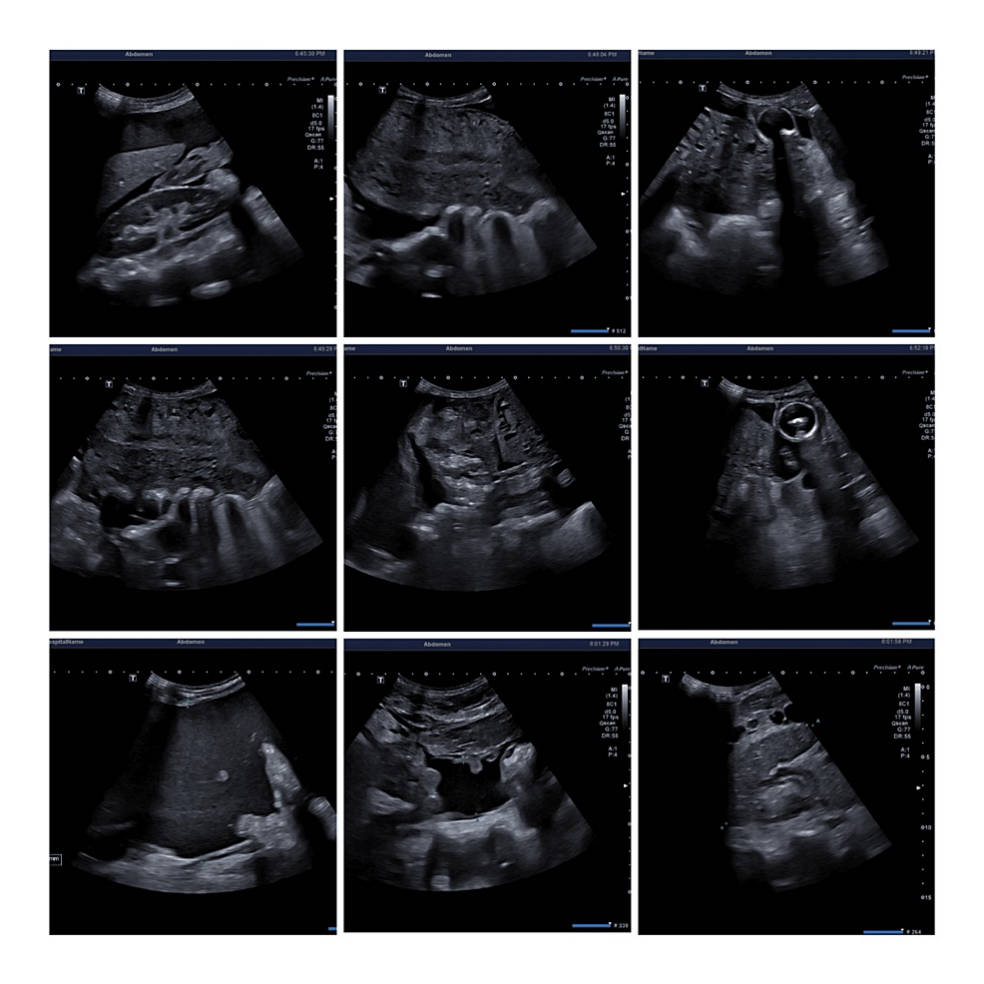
Ultrasound images of the abdomen
